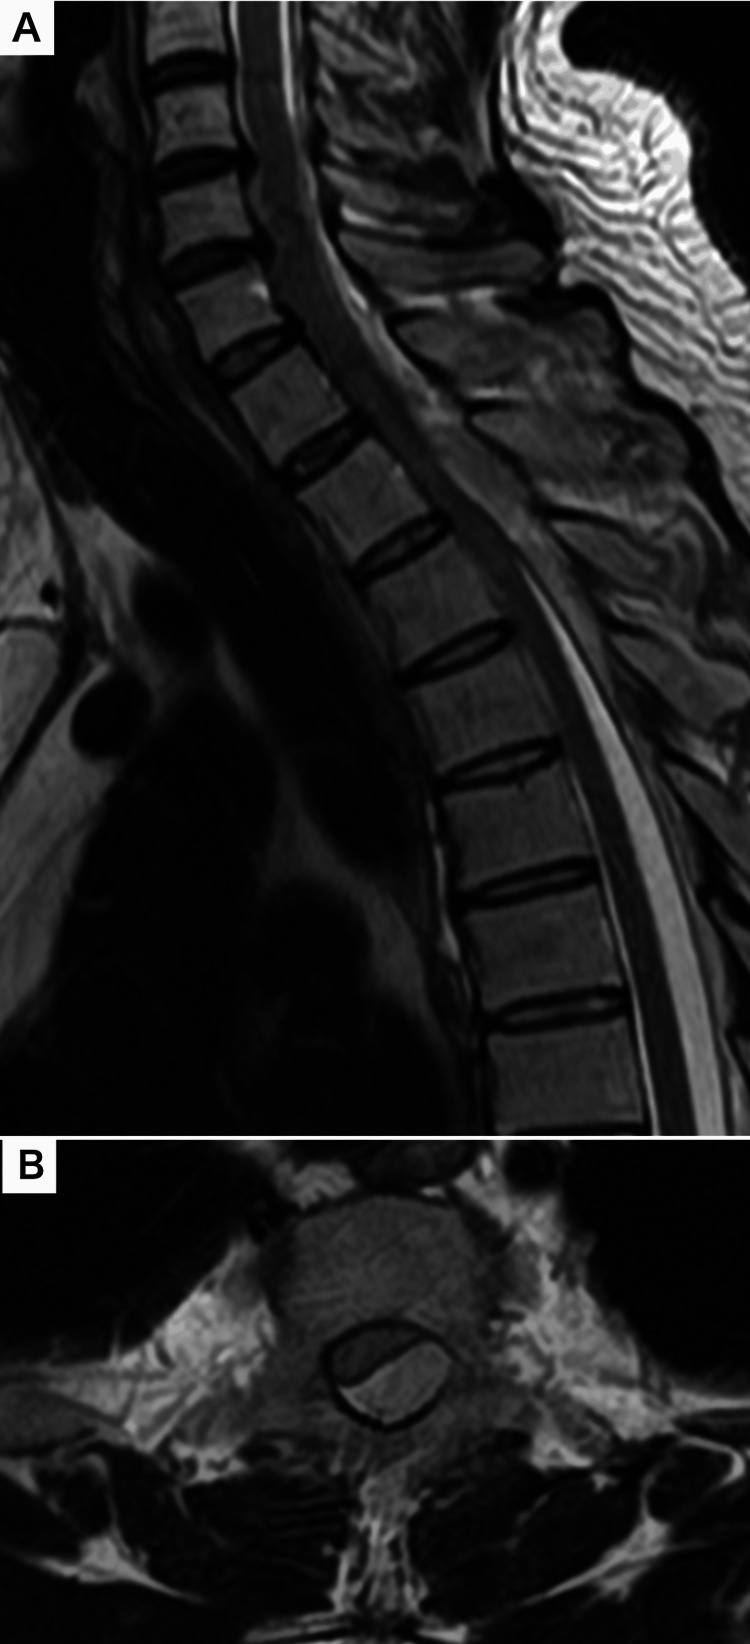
Thoracic spine MRI (T2-weighted image) Sagittal (A) and axial (B) T2-weighted MRI of the spine confirms the acute subdural hematoma, which appears as a hyperintense collection causing significant cord compression.
